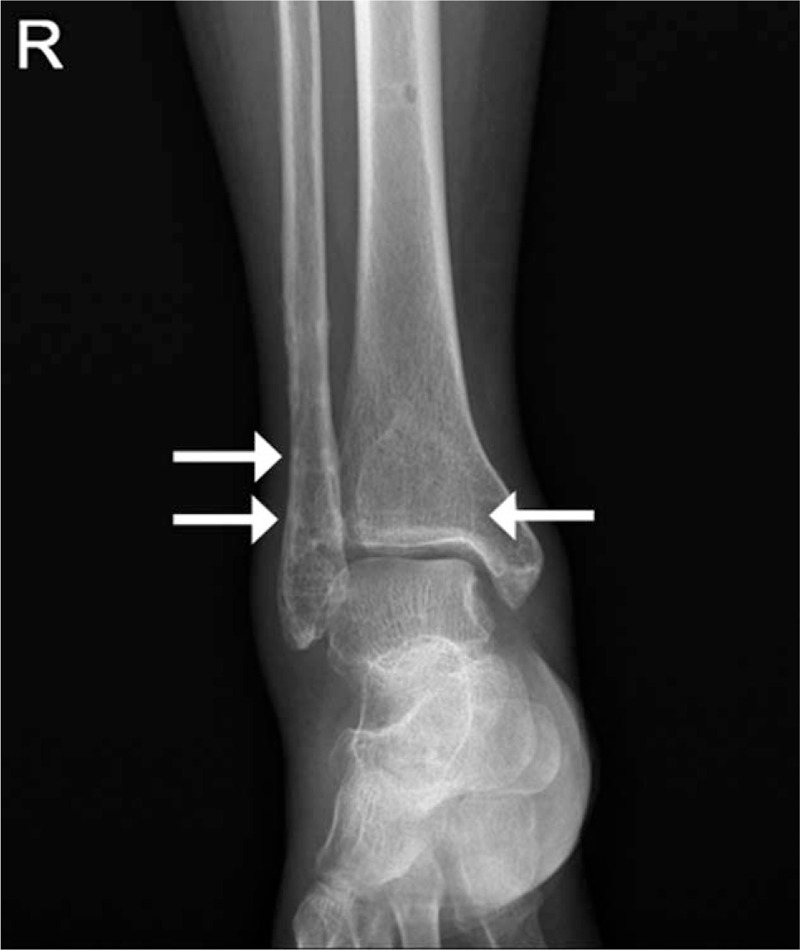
Plain ankle radiographs. Anteroposterior view. Previous operative evidence of along the right tibia and fibula (arrows).
